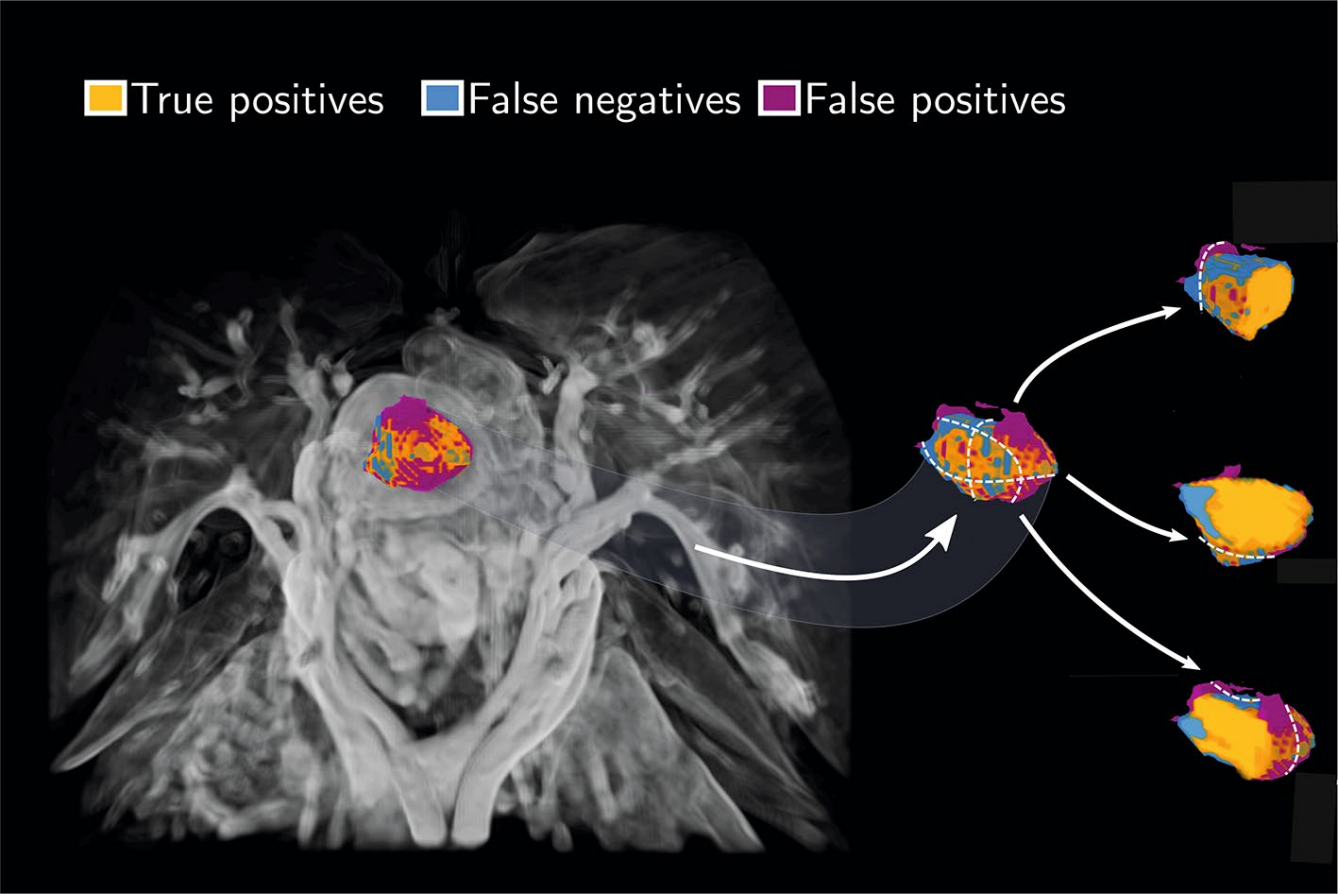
Volume rendering (left panel) of manual and automated ML segmentation of the primary tumor from one patient in terms of true positives (orange), false negatives (blue), and false positives (purple). The 3D segmentation is shown along with the gradient-enhanced 3D volume rendering of the T1-weighted VIBE sequence as a background image. Furthermore, three separate paraxial slices from the same data set are depicted together with a close-up view of the tumor and outlined segmentations (right panel). A majority of the false predictions occur in the tumor periphery, indicated with blue and purple labels. The white, dotted lines are the three orthogonal cutting planes used for creating the corresponding slicing plot.
